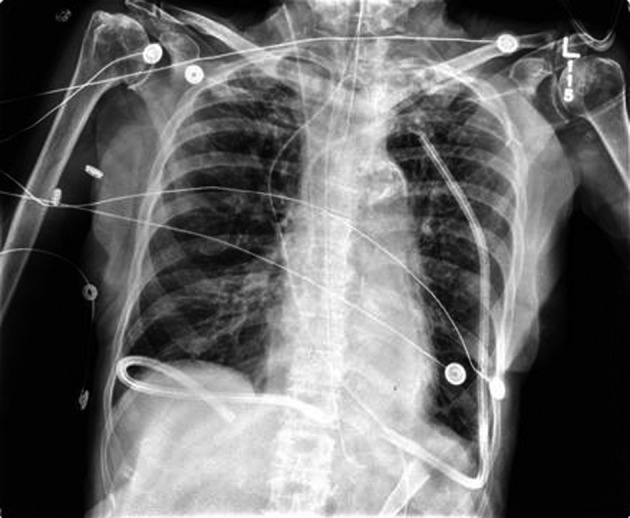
Immediate Post-operative Chest X-Ray following THE.
